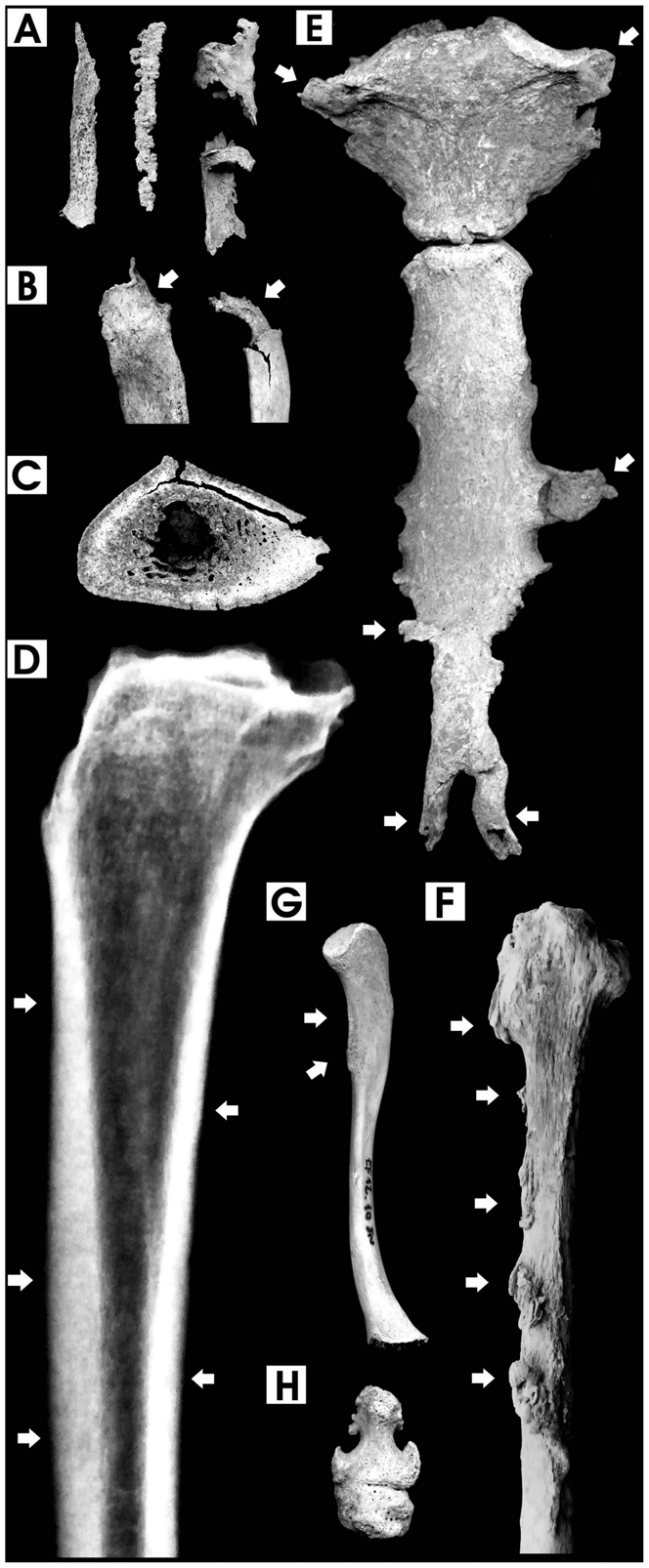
Pathological features in chest and long bones. A. Calcified ligaments and interosseous costal cartilages, 40-year-old male; B. Proximal first and sixth rib epiphyses with prominent exostoses due to interosseous cartilage calcification, 27-year-old female and 40-year-old male; C. Cross section of the mid-shaft of tibia showing extensive cortical thickening, increased bone matrix density, intracortical resorption and reduced medullary space, 40-year-old male; D. Digital x-ray image (lateral view) of the previous tibia, showing a “marble-like” appearance (arrows) symptomatic of marked osteosclerosis; E. Prominent calcification of costosternal and costoxiphoid ligament attachments (arrows) in the sternum, 40-year-old male; F. Ligamentous and interosseous membrane ossification at multiple sites (arrows) in the fibula, 40-year-old male; G. Calcification and osteophytosis at the attachment of the deltoid muscle (arrows) in the clavicle, 9-year-old male; H. Ankylosis of toe distal interphalangeal joint, 29-year-old male (bone images are in 1∶2 size).
